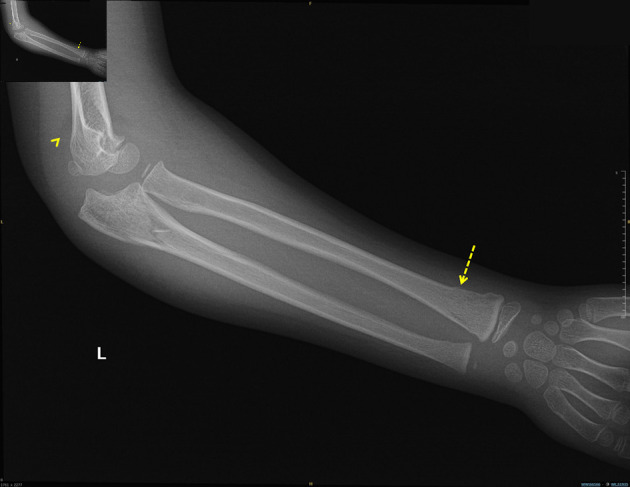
Lateral elbow radiograph demonstrating the posterior fat pad sign (arrowhead) and distal radial fracture (dashed arrow).
